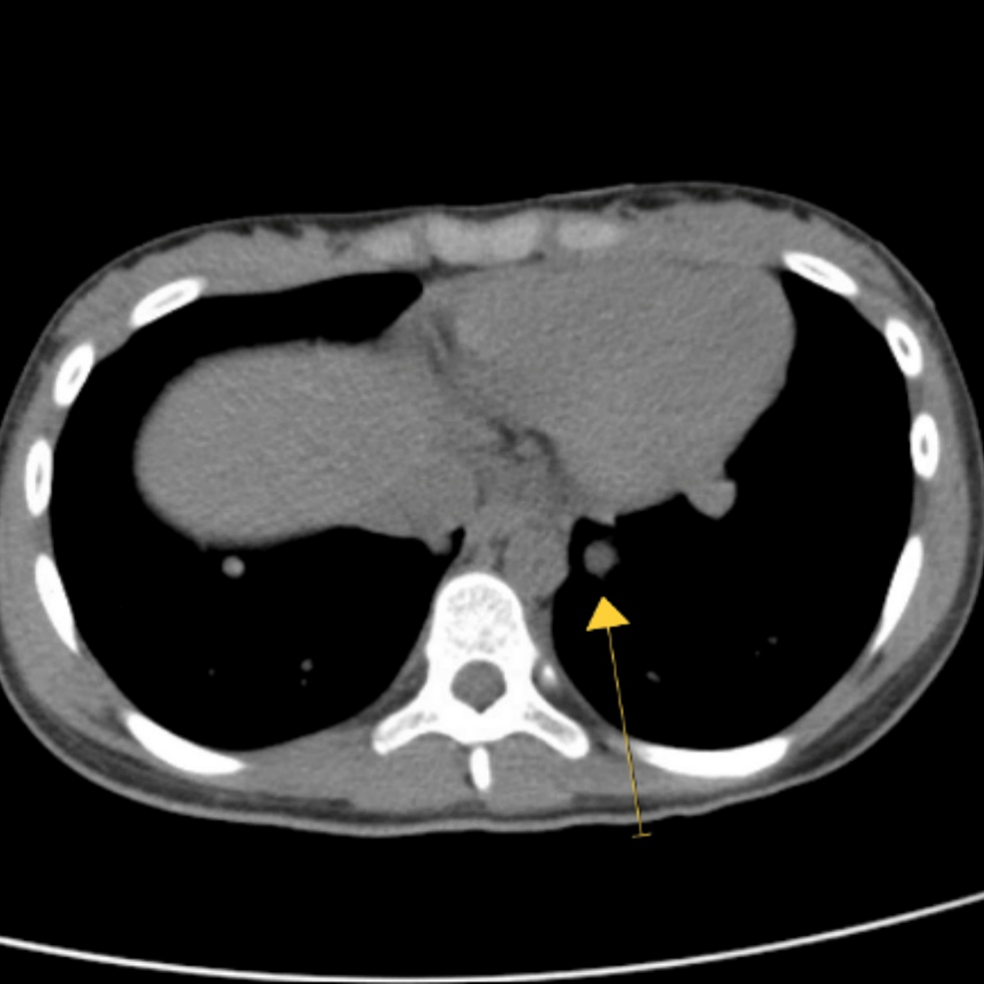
Chest CT scan of the patient Yellow arrow points to multiple bilateral pulmonary nodules measuring up to 1 cm in the left lower lobe
